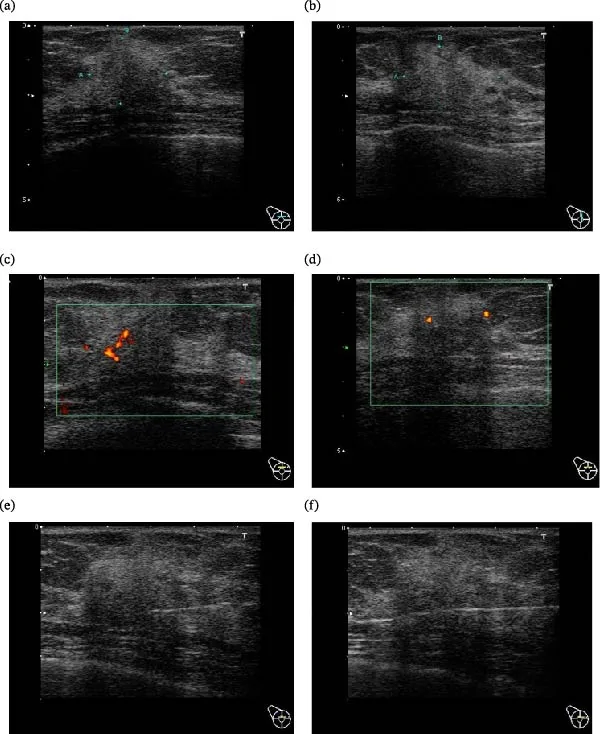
Breast ultrasonography (a, b, c, d, e, f). Breast US revealed a heterogeneous mass measuring ~5 cm with an irregular margin and ill‐defined border in the upper‐outer and upper‐inner quadrants of the right breast, respectively, which was diagnosed as a category 5 lesion (a, b). Color Doppler echo imaging showed a hypervascular pattern (c, d). CNB was performed (e, f).
